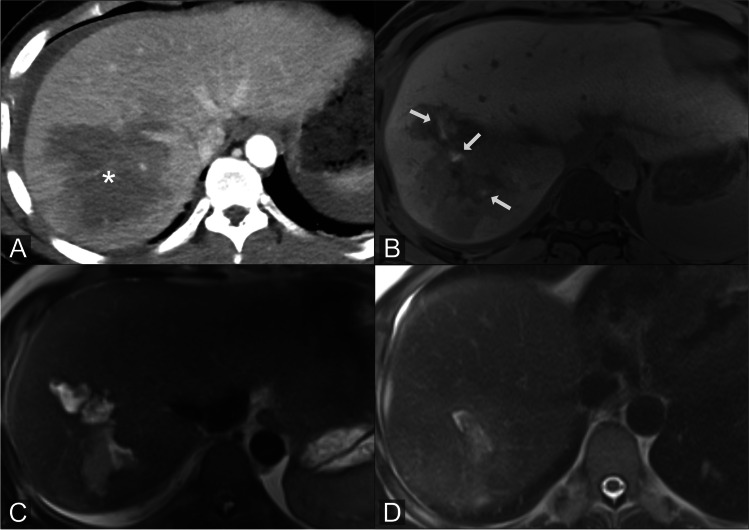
Intraparenchymal bile leakage: 32-year-old patient admitted to our hospital with blunt liver trauma. Initial CT scan shows laceration of the right liver lobe (A). Gd-EOB-DTPA-MRCP performed 4 days later shows intraparenchymal BL with Gd-EOB-DTPA extravasations (arrows) (B). T2w image shows the extent of the parenchymal lazeration (C). MRI follow-up 5 weeks after initial trauma: T2w image shows significant decrease of intrahepatic lazeration (D). No further intervention was required
